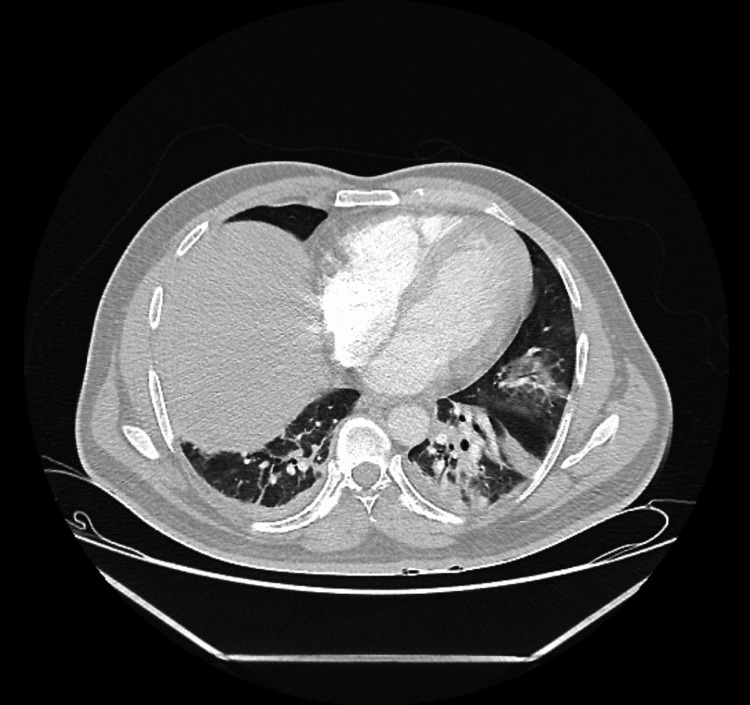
CT scan of the chest showing left lower lobe consolidation.
